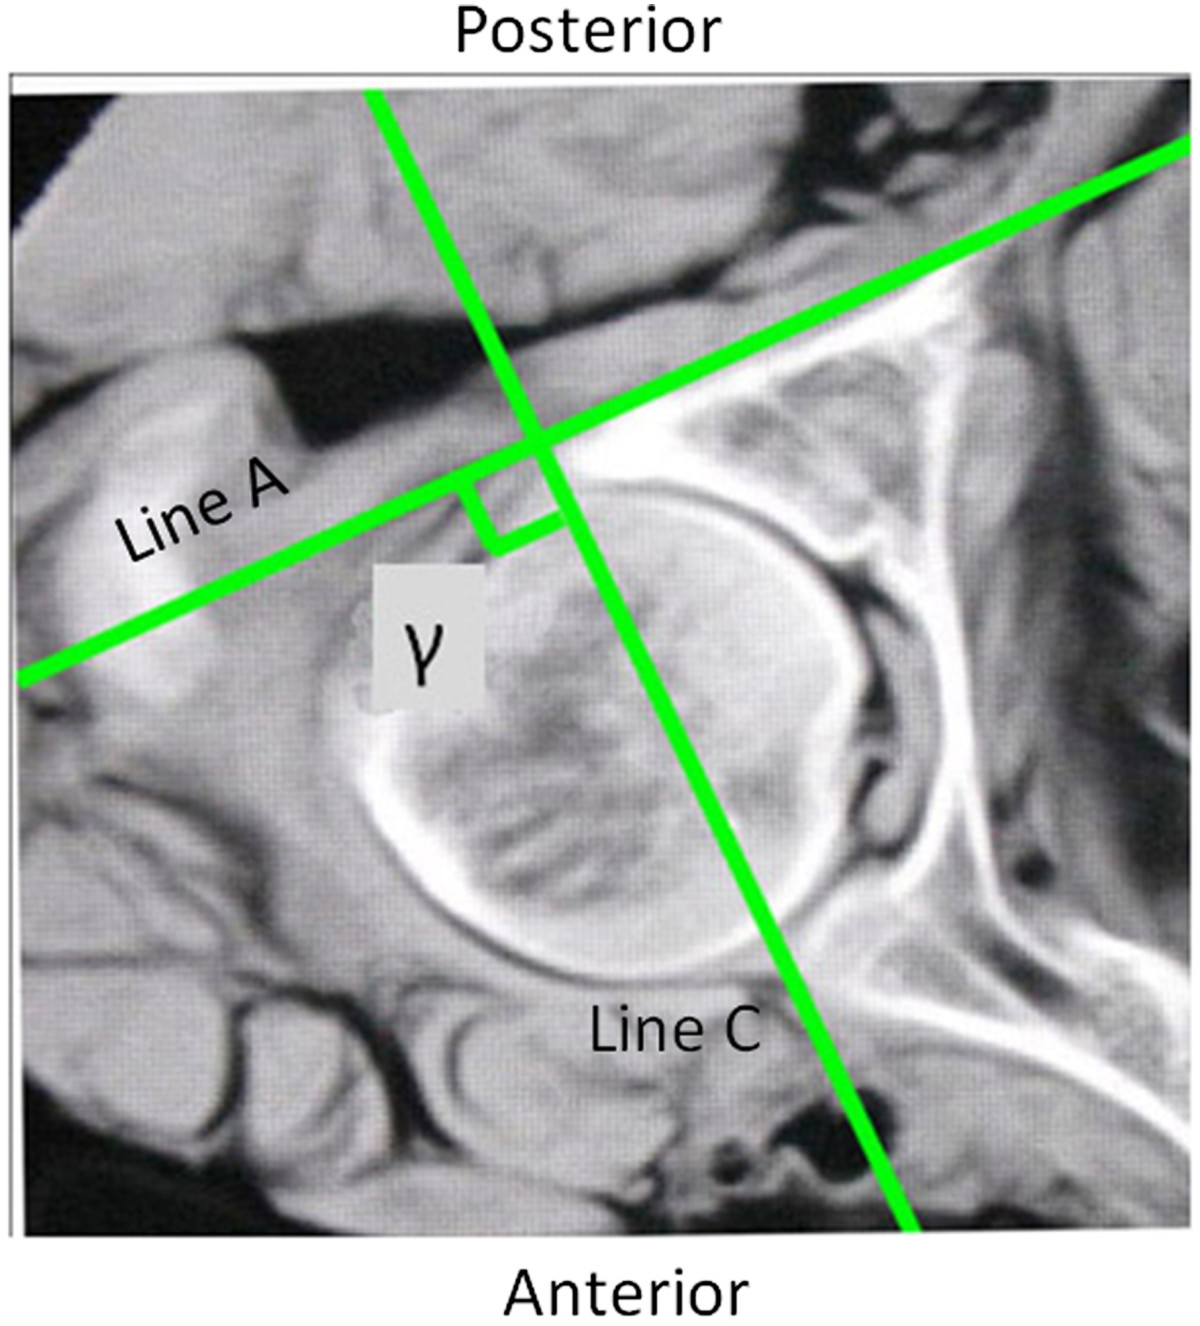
On the axial CT images, Line C represents the line running through the anterior and posterior points of the lateral brim of the acetabulum. The angle between Lines A and C is labelled angle γ.
